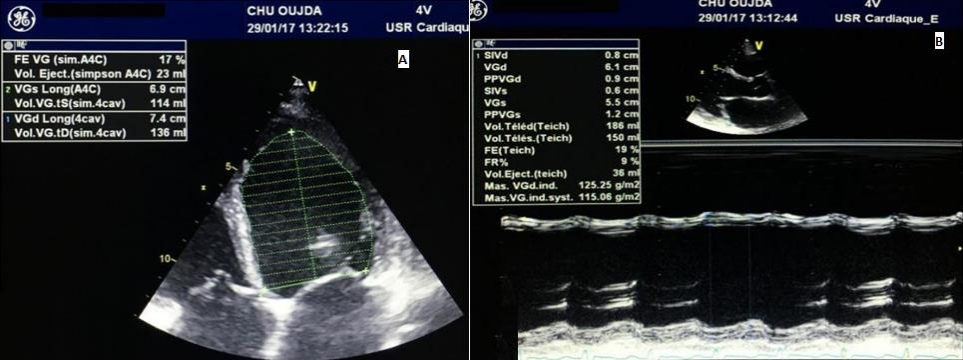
A) transthoracic echocardiography (TTE) in apical 4-chamber, 2-dimensional echocardiogram showing severe systolic dysfunction at 17%; B): M-mode transthoracic echocardiography on long-axis view showing dilated LF with LVEDD of 61 mm and severe systolic dysfunction
